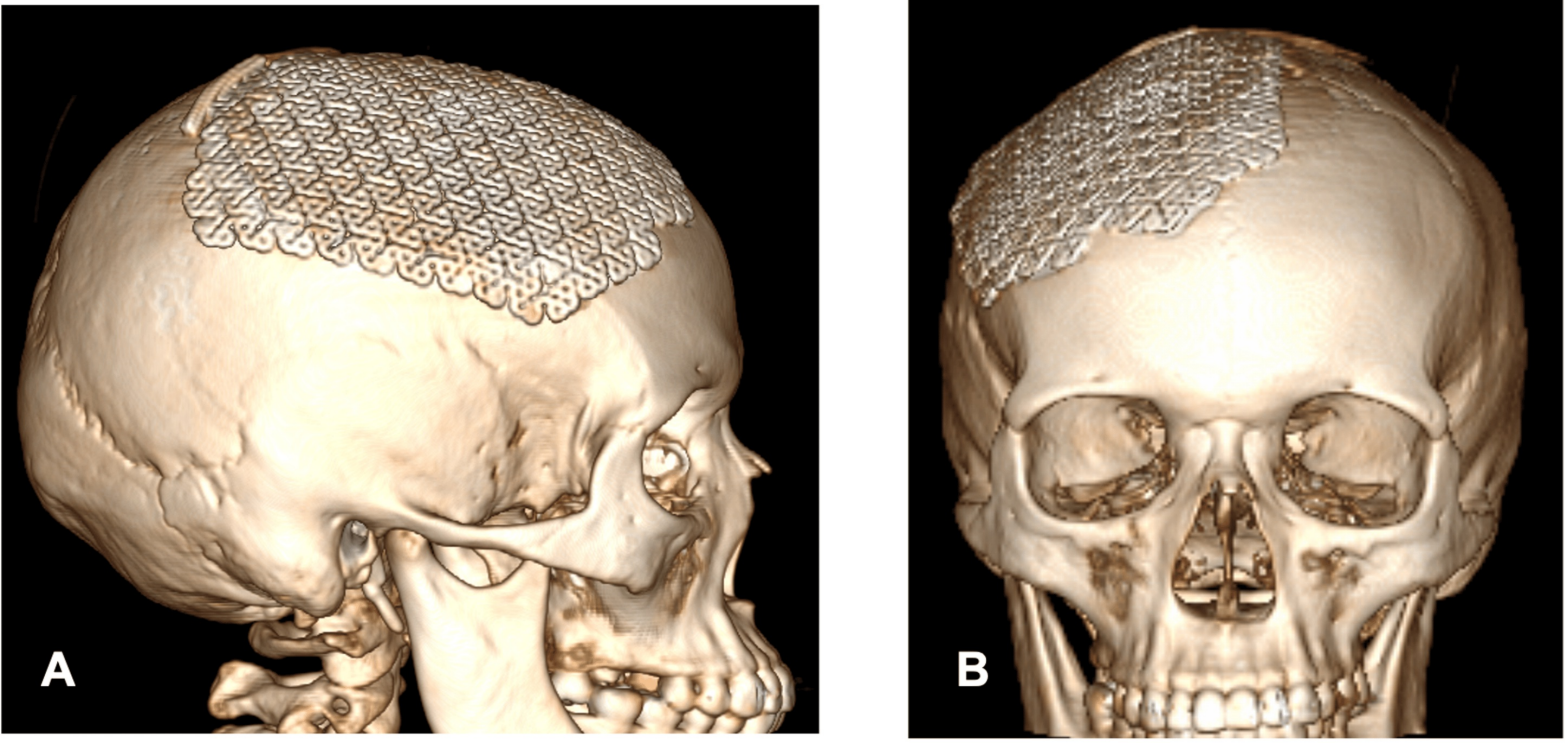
A 3D reconstruction of postoperative CT scan following cranioplasty with low-profile titanium mesh for the correction of cranial defect while preserving the patient's anatomy as observed above. Symmetry was achieved to look as the contralateral side. A. Sagittal view. B. Coronal view.
